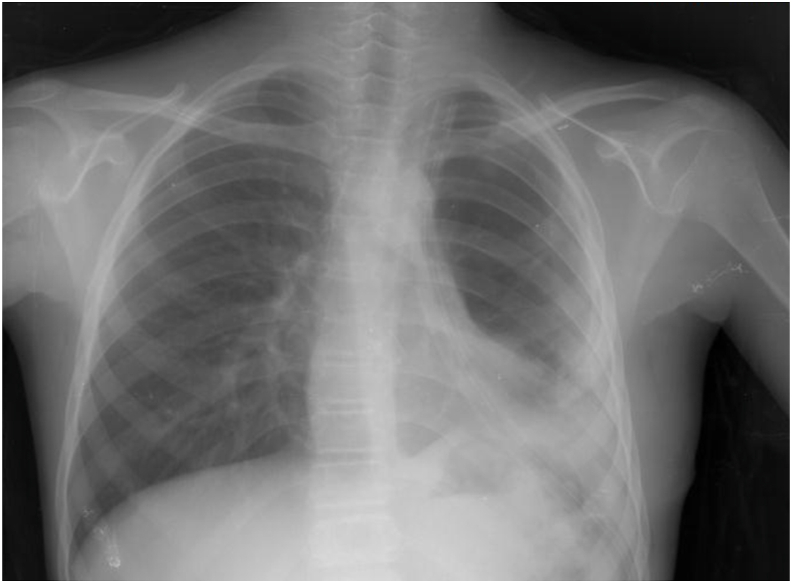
Normal chest X-ray four days after the surgery.
